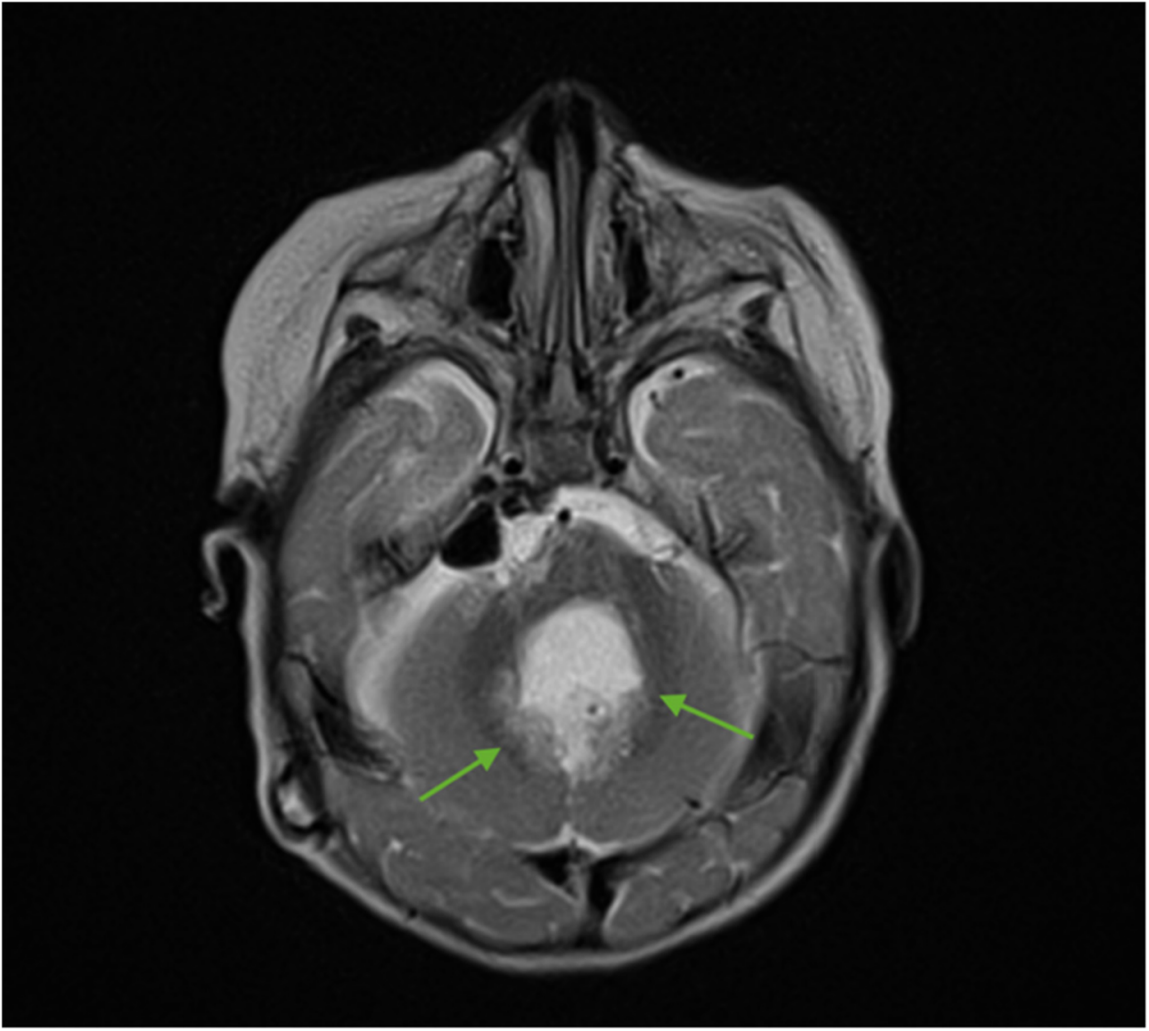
Brain MRI (axial T2 + C). Postoperative changes, pathologic contrast accumulation in the walls of the resection cavity, and residual tumor in the vermis (green arrows).
